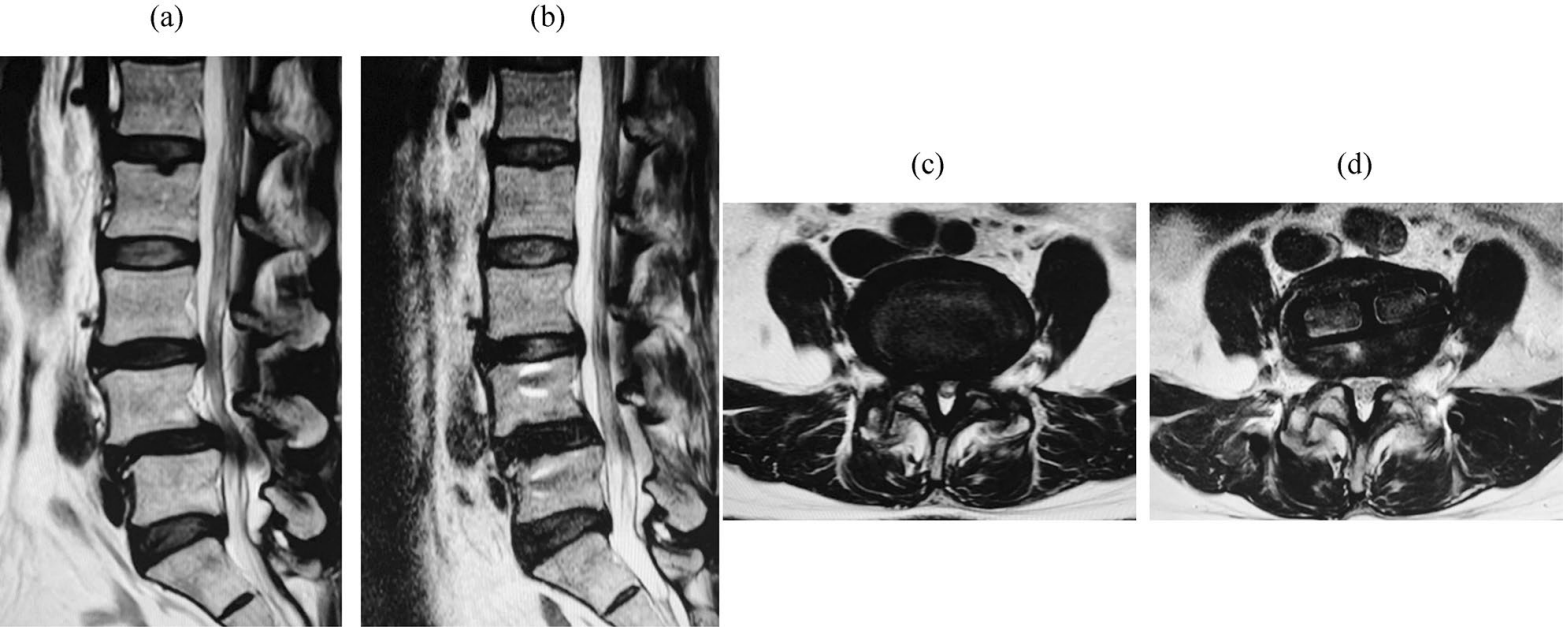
Images of a 54-year-old female patient with L4 spondylolisthesis. (a) Preoperative sagittal MRI image. (b) Postoperative sagittal MRI image. (c) Preoperative axial MRI image at the L4–5 disc level. (d) Postoperative axial MRI image at the L4–5 disc level 12 months after XLIF with PPS fixation (IDP) surgery. Pain improved from preoperative to postoperative measurements: back pain, NRS-BP from 9 to 0; leg pain, NRS-LP from 0.5 to 0; and leg numbness, NRS-LN from 9 to 0. MRI, magnetic resonance imaging; IDP, indirect decompression; NRS, numeric rating scale; NRS-BP, NRS for low back pain; NRS-LP, NRS for leg pain; NRS-LN, NRS for leg numbness.
